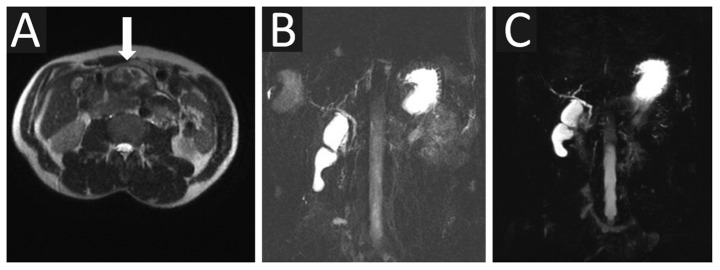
Imaging examination performed on a 35-year-old male diagnosed with pancreatic pseudocyst. (A) T2-weighted imags revealed that the pancreatic pseudocyst exhibited low signal intensity (arrow). (B) 2D MRCP did not reveal the cystic lesion. (C) 3D MRCP exhibited the superior image quality compared with 2D MRCP, but did not reveal the cystic lesion and pancreatic main duct. 2D, two-dimensional; 3D, three dimensional; MRCP, magnetic resonance cholangiopancreatography.
